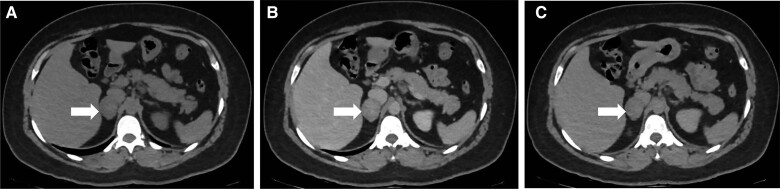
Axial images from an unenhanced and contrast-enhanced computed tomography scan showing a lipid-poor 35 × 27 mm right adrenal mass (arrows). A) Unenhanced attenuation of 40 Hounsfield units (HUs). B) Contrast-enhanced venous phase attenuation of 90 HUs. C) Delayed contrast-enhanced attenuation of 65 HUs.
